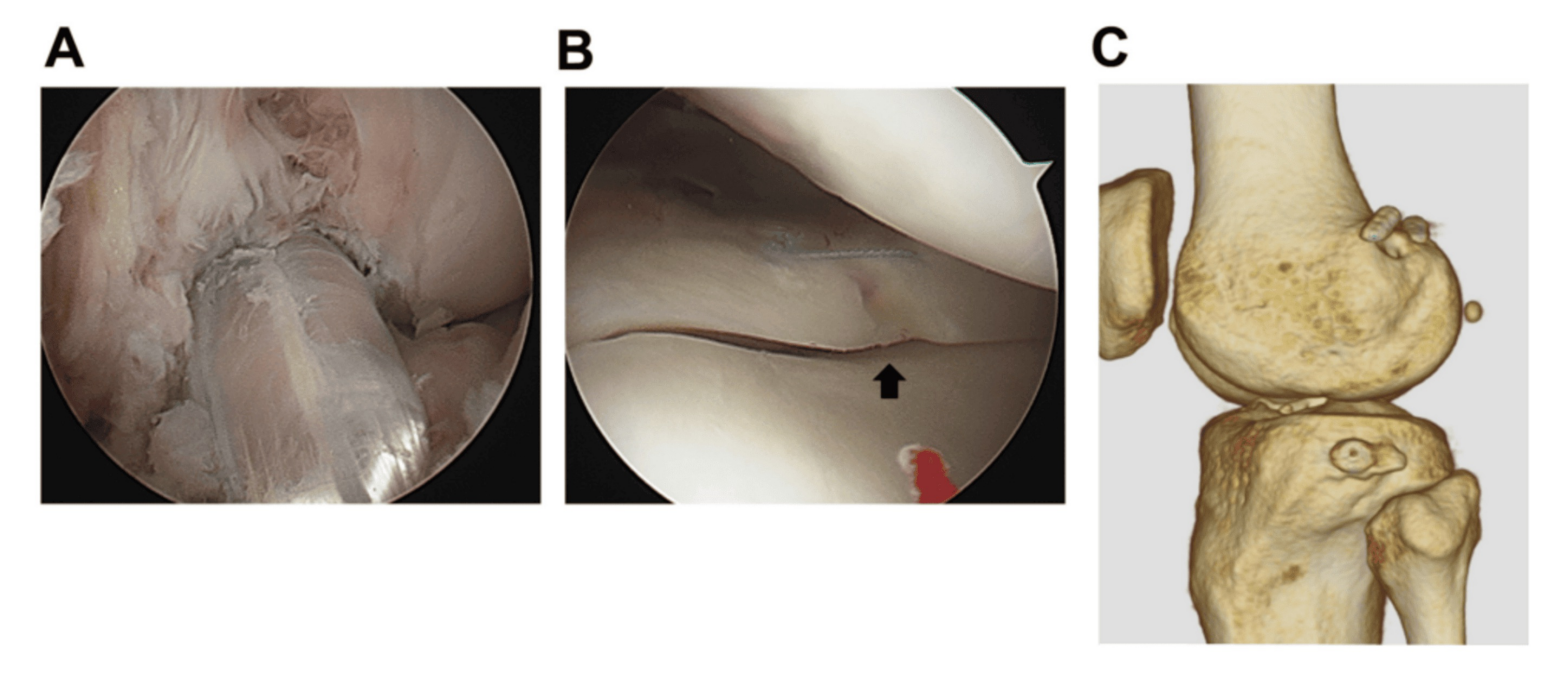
Arthroscopic view images at the time of ACL reconstruction and the postoperative CT image. (A) After the double-bundle ACL reconstruction with a hamstring tendon (anteromedial portal view). (B) The repaired site of the lateral meniscus (black arrow). (C) The postoperative three-dimensional CT image. ACL: anterior cruciate ligament; CT: computed tomography
